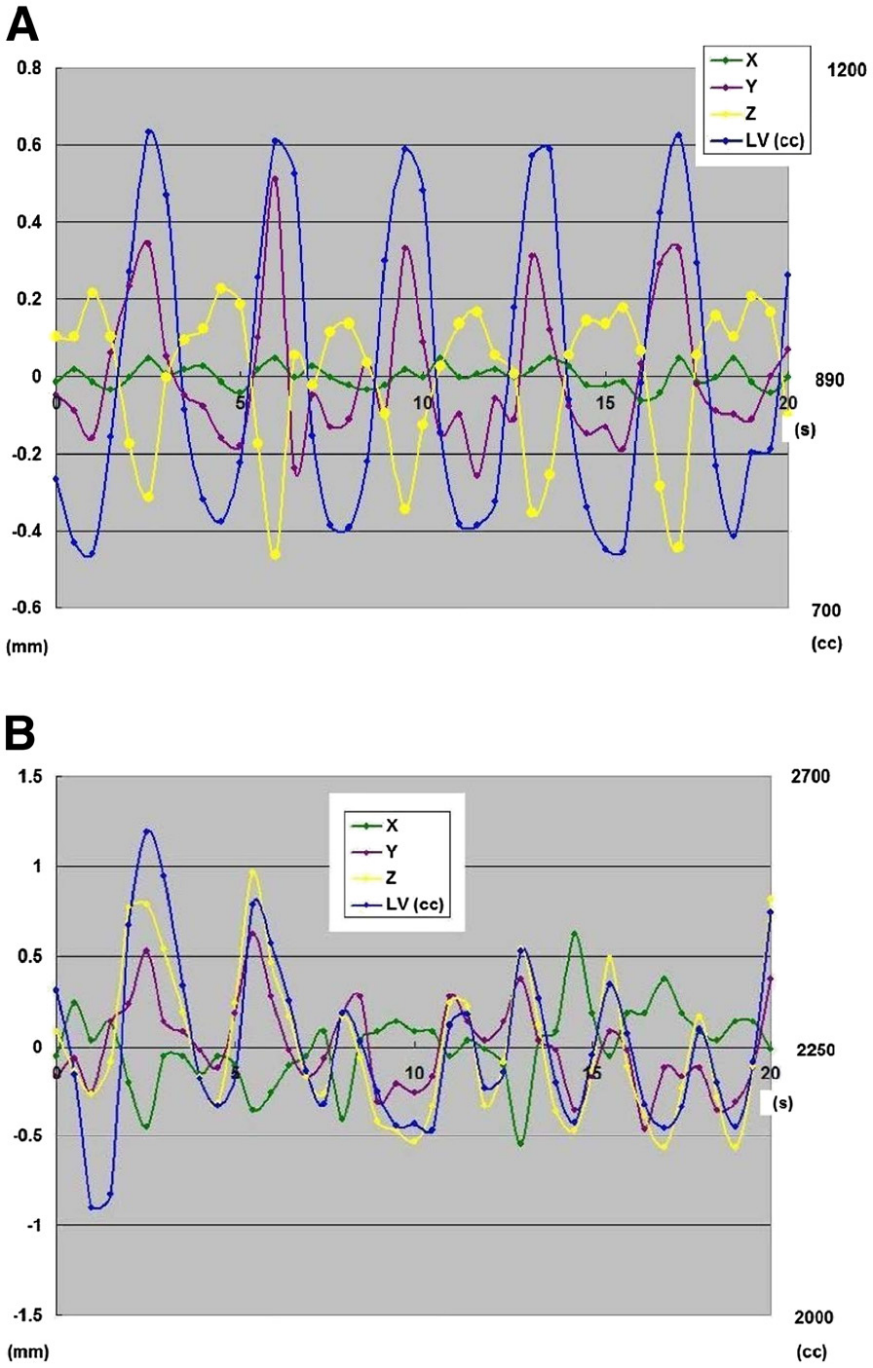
Centroid trajectories of metal clips in all 41 phases for 20 s of the four-dimensional CT scans. (A) Patient 6. (B) Patient 1. RL = right–left; AP = anterior–posterior; CC = cranio–caudal.
